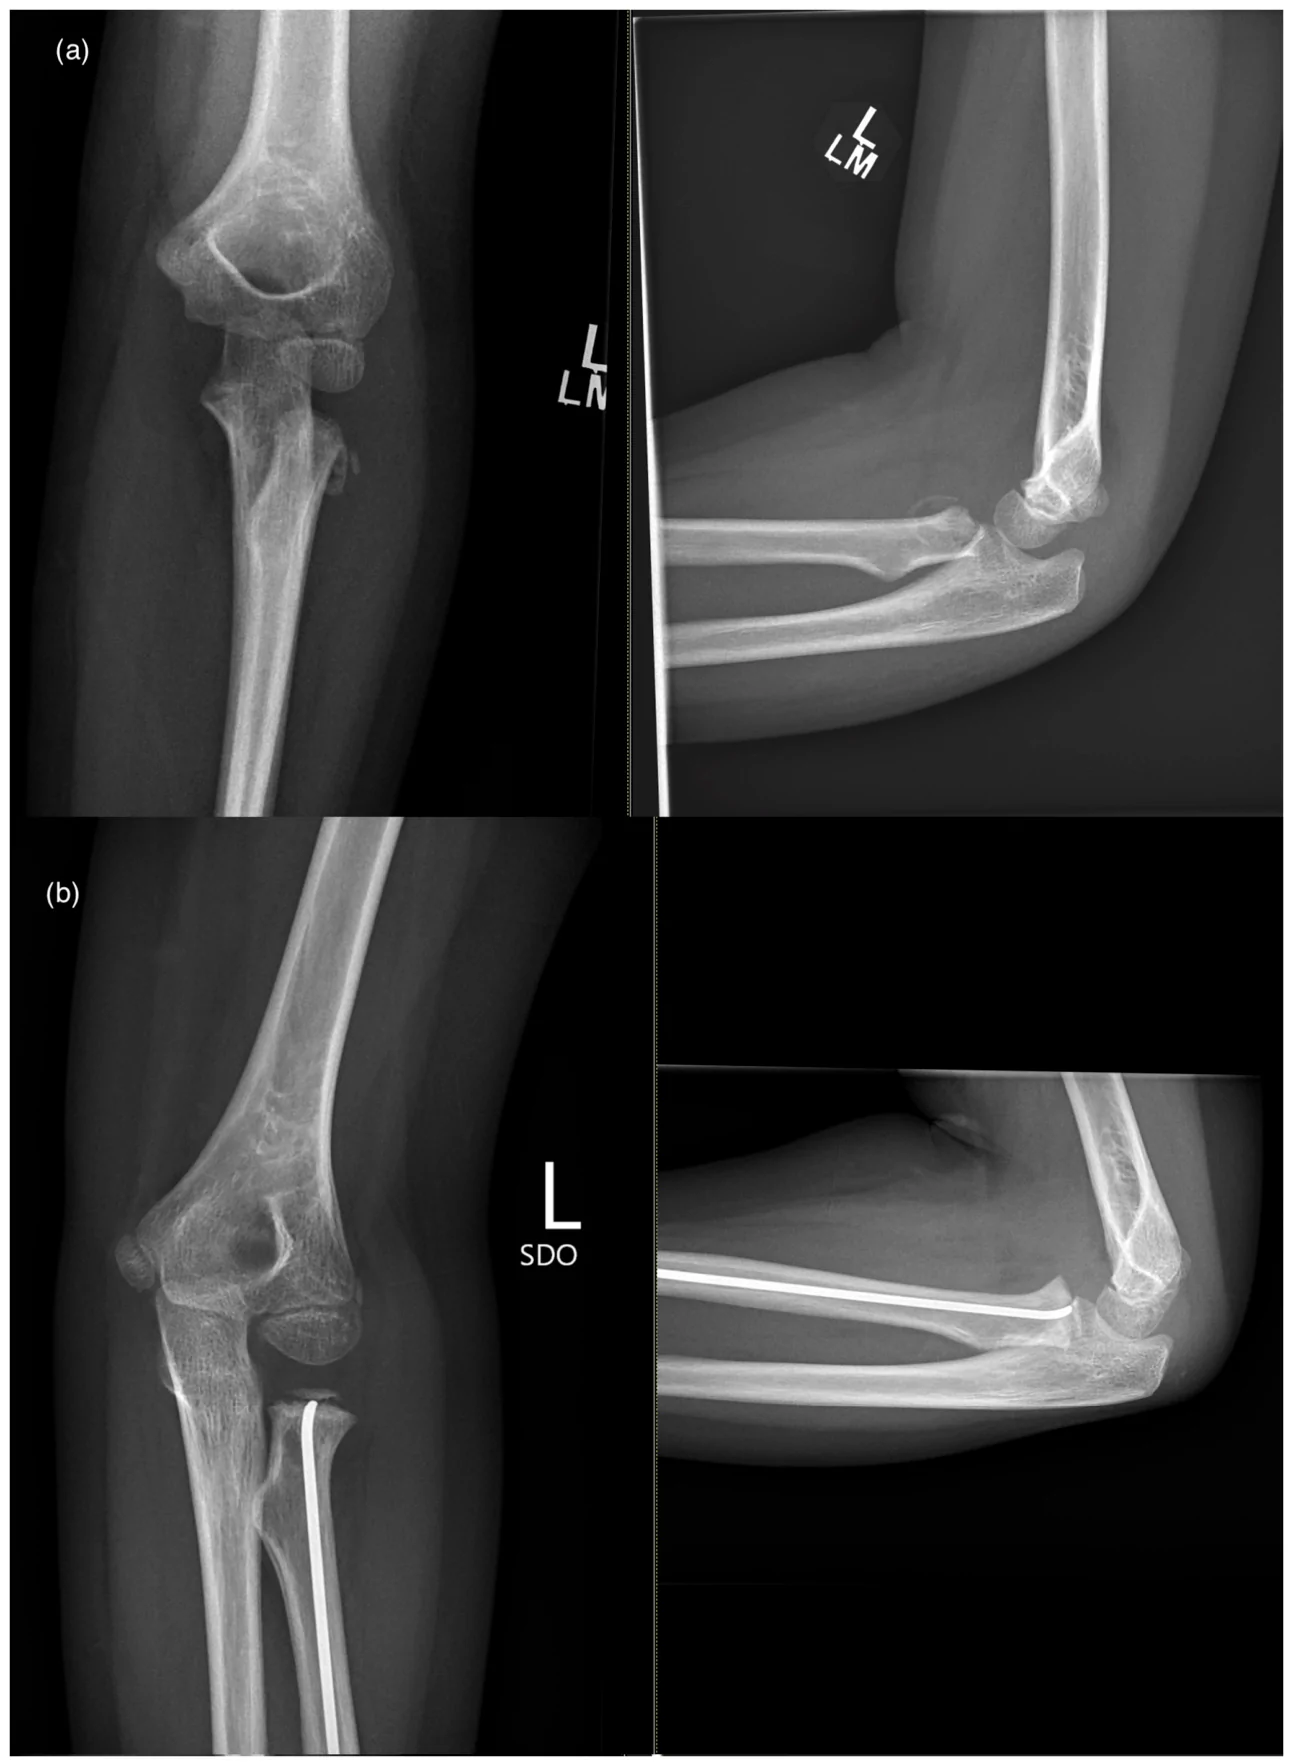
Radiographs depicting a displaced radial neck fracture; (a) preoperative radiographs; and (b) postoperative radiographs depicting a united fracture following the subcapital realignment procedure.
